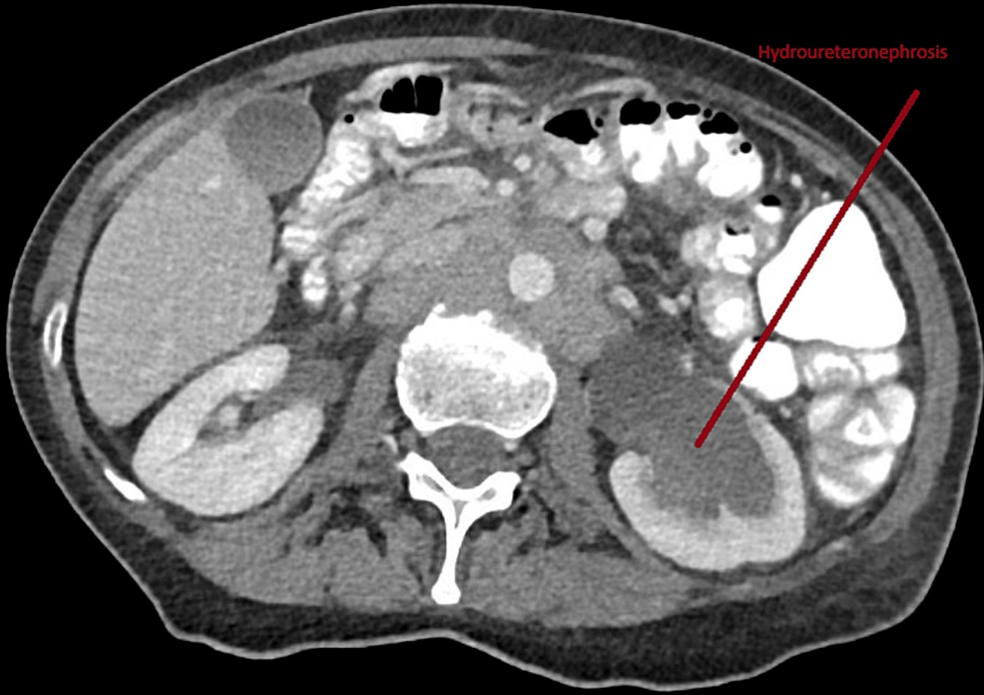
CT abdomen The uterus is enlarged and heterogeneous in appearance with a much larger round heterogeneous mass lesion in the superior aspect of the uterus, 7.1 x 6.0 x 6.8 cm. Extensive periaortic/retroperitoneal bulky lymphadenopathy, encasing the infrarenal abdominal aorta and extending to the bilateral iliac chains. Severe LEFT hydronephrosis and LEFT hydroureter secondary to extrinsic compression on the distal LEFT ureter secondary to the pelvic mass.
